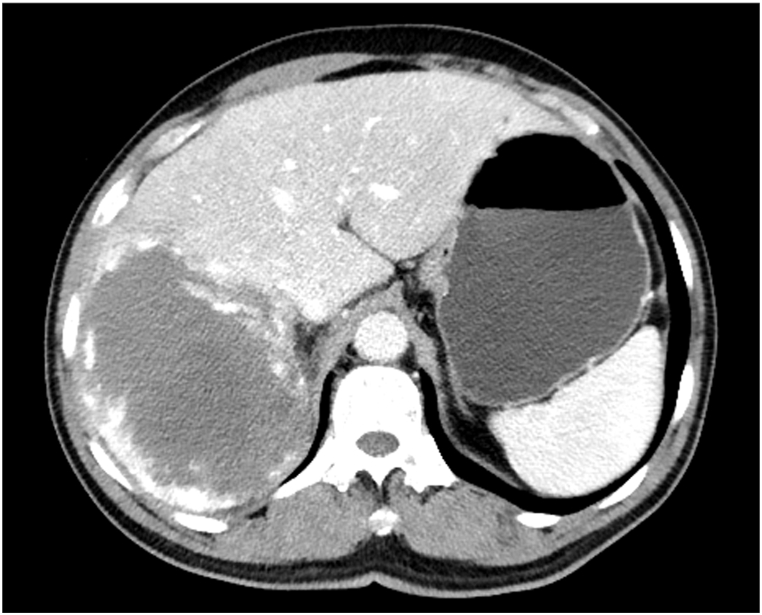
A 1.1-month follow-up CT demonstrated reduction in CHL size to 106.7 × 141.3 mm with residual enhancement in the periphery of the CHL.
